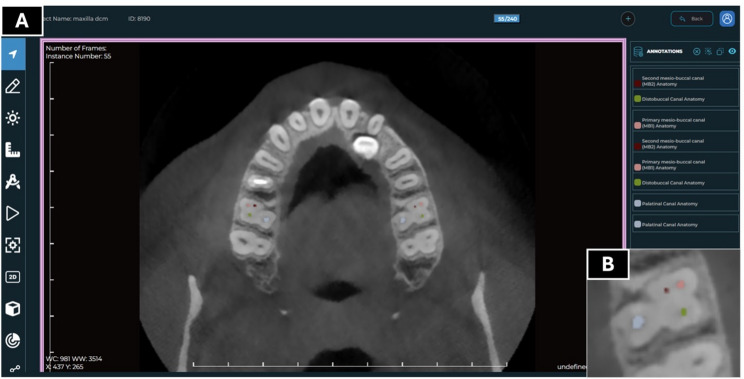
On the labeling module: (A) Labeling of MB2 and main root canals in a 3D CBCT image; (B) A magnified axial section example showing the labeled MB2 and main root canals
